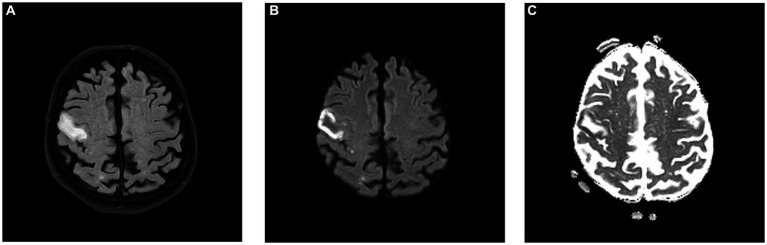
Brain MRI. Acute ischemia of the right precentral gyrus. (A) FLAIR transverse image, (B) DWI transverse image, (C) ADC transverse image.
